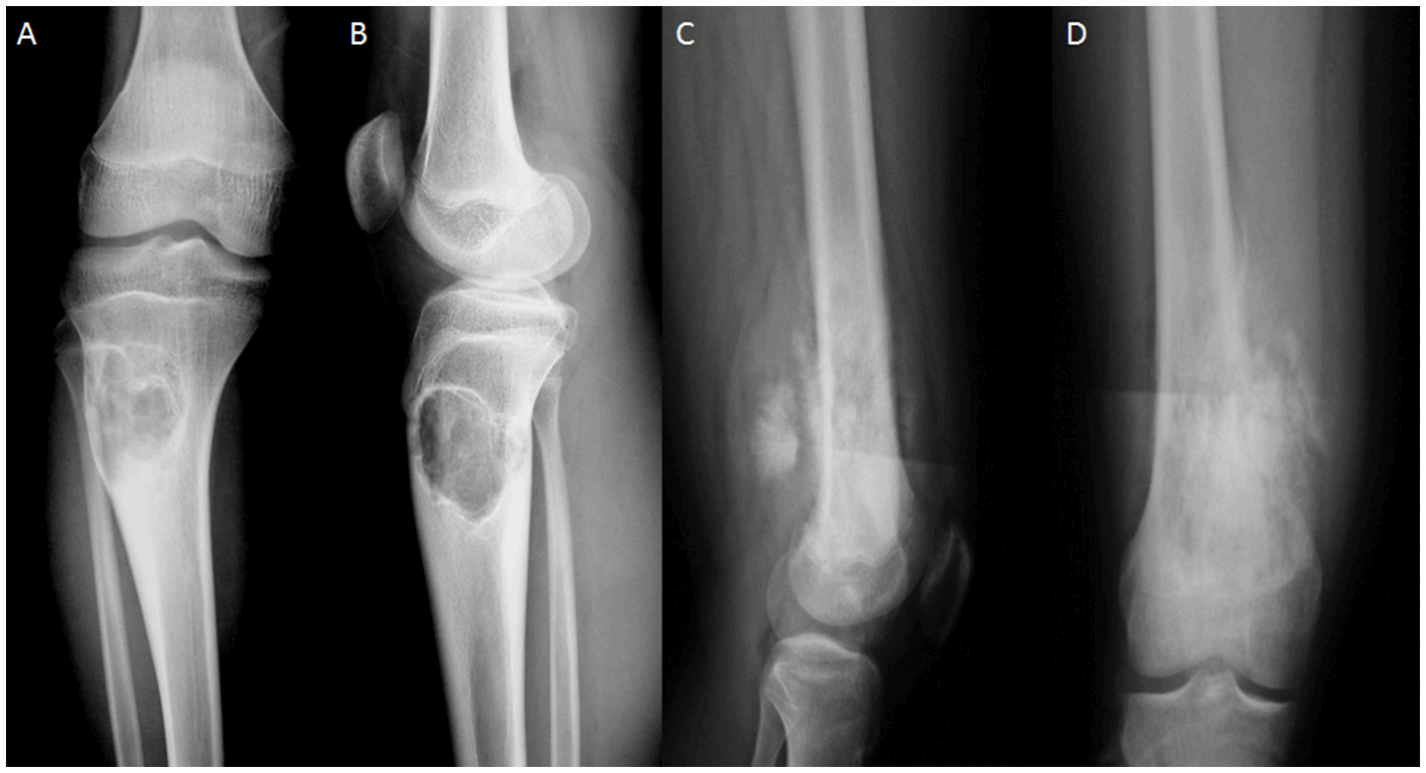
MRI images of OS at different stages. (A) OS at G1 grade. (B) OS at G2 grade. (C) OS at G3 grade. (D) OS at G4 grade.
